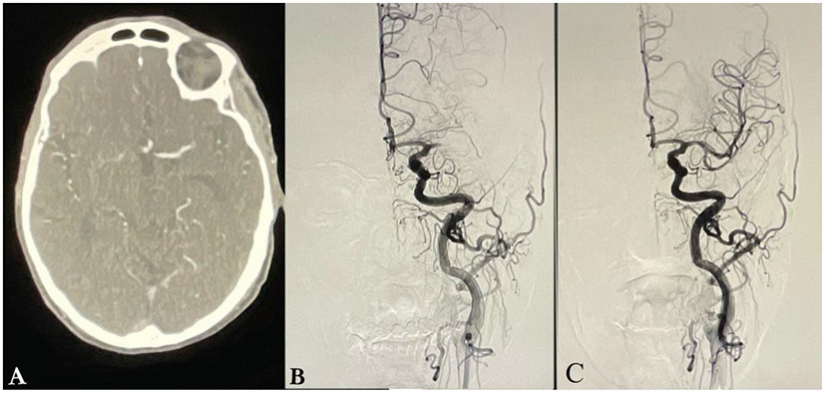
Imaging of a patient without a “Tilted-V Sign.” (A) Axial images of CT angiogram of head showing lack of flow beyond the occluded M1-Middle Cerebral Artery (MCA) segment without filling of proximal M2 segments of the middle cerebral artery (MCA). (B) Digital subtraction angiography (DSA) before endovascular thrombectomy (EVT) showing complete occlusion of the M1-MCA segment but without visualization of vessels distal to the occlusion. (C) DSA following EVT showing successful recanalization.
